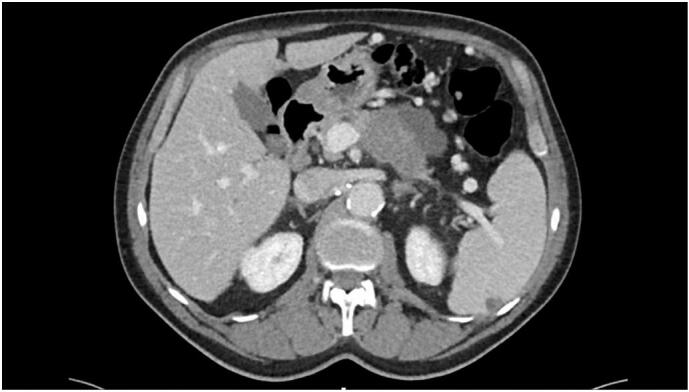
Patient 1 abdominal CT-scan.
